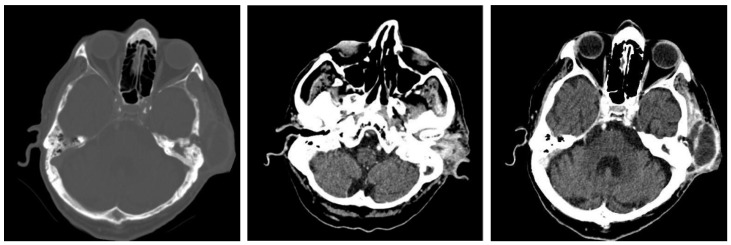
Axial CT sections, bone and parenchymal window—extensive area of osteolysis in the left temporal bone and external wall of the mastoid, absence of pneumatization of left-sided mastoid cells and reduced pneumatization of right-sided mastoid cells, epididymal collection, diffuse infiltrative appearance of the preauricular soft tissues.
